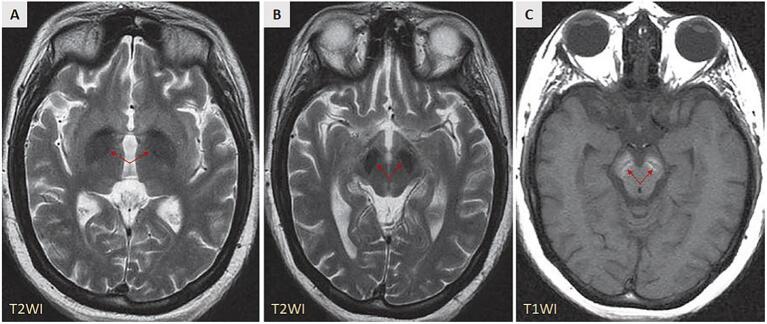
[A] Axial T2-weighted MR image at level of basal ganglia demonstrates hypointensity involving bilateral globi pallidi (*arrows*). [B] Axial T2-weighted MR image at level of substantia nigra demonstrates hypointensity involving substantia nigra and subthalamic nuclei on both sides (*arrows*). [C] Axial T1-weighted MR image at level of substantia nigra demonstrates bilateral symmetric, high signal involving the substantiae nigrae, with a central band of hypointensity (*arrows*).
